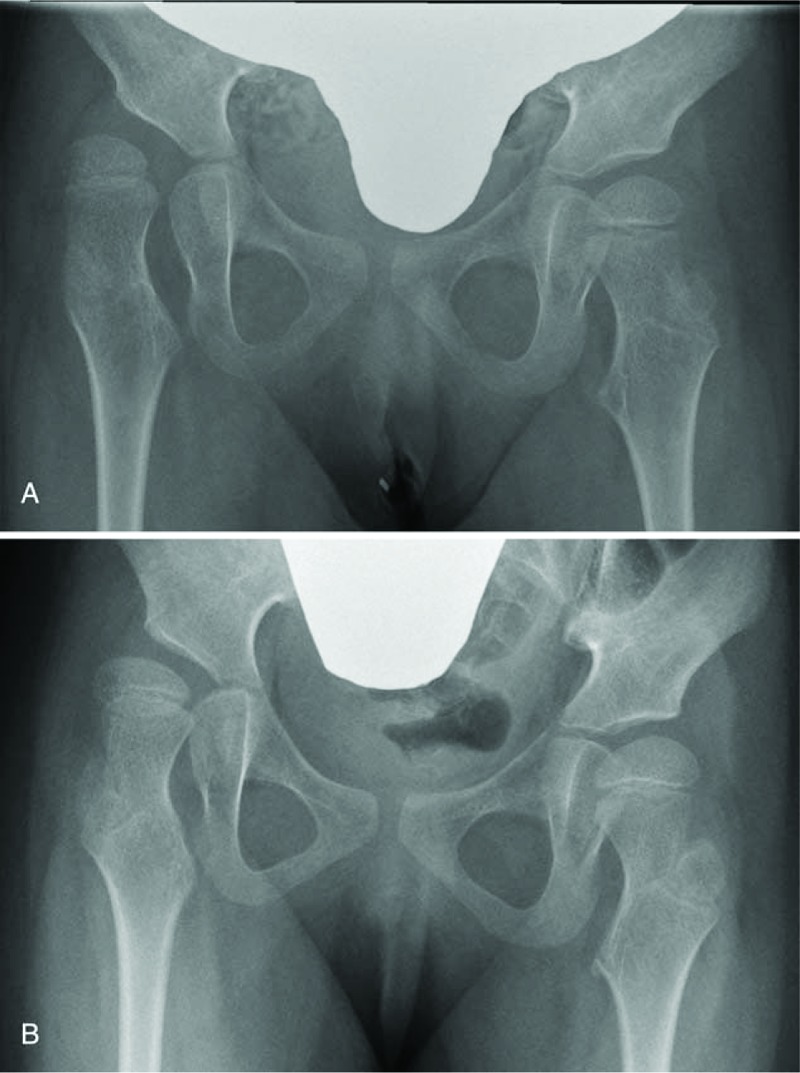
Radiographic representation of a patient with poor prognosis before and after the soft tissue release surgery. Radiographs showing the hips of a girl with cerebral palsy, Gross Motor Function Classification System (GMFCS) level V. A, At 3 years of age with a migration percentage (MP) of 62% on the right side and 24% on the left side, acetabular index (AI) of 37.1° on the right side and 20.6° on the left side. B, 3 Years after soft tissue release, MP was 41% on the right side and 26.7% on the left side, with AI of 35.3° on the right side and 18.3° on the left side.
